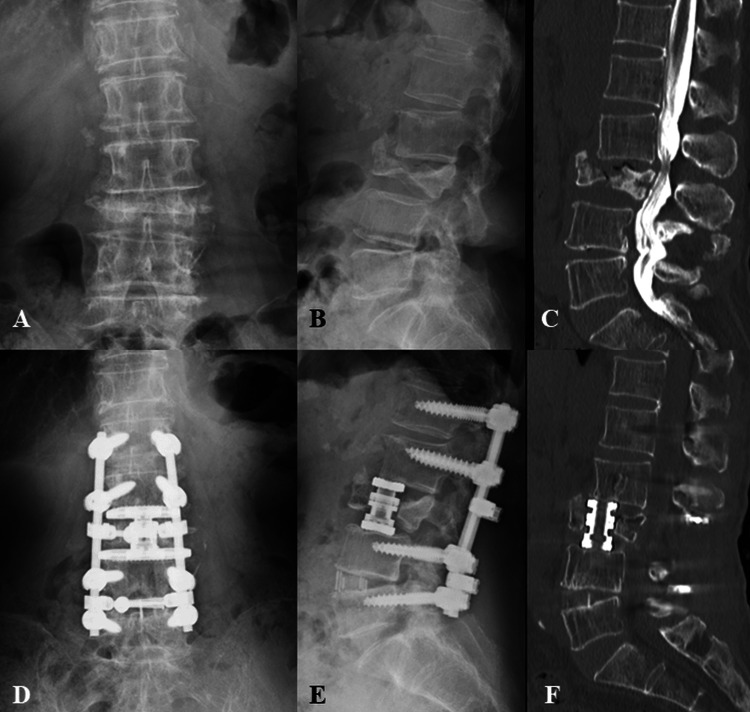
LP approach Representative case of the LP approach. An 84-year-old female patient who underwent the LP approach for L3 osteoporotic vertebral collapse. Preoperative posteroanterior (A) and lateral (B) radiographs and sagittal CT myelography (C). Postoperative posteroanterior (D) and lateral (E) radiographs, and CT sagittal image (F). CT: computed tomography, LP approach: lateral-posterior combined approach
